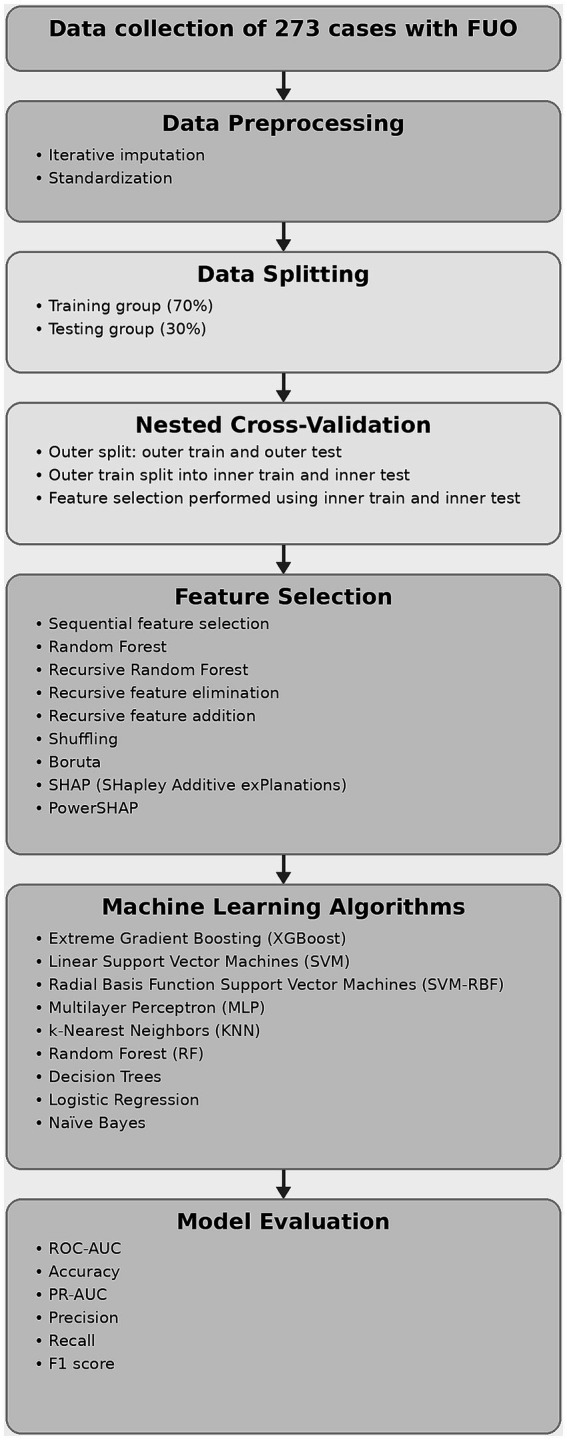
Overview of the machine learning framework for predicting PET/CT requirement in FUO patients.
